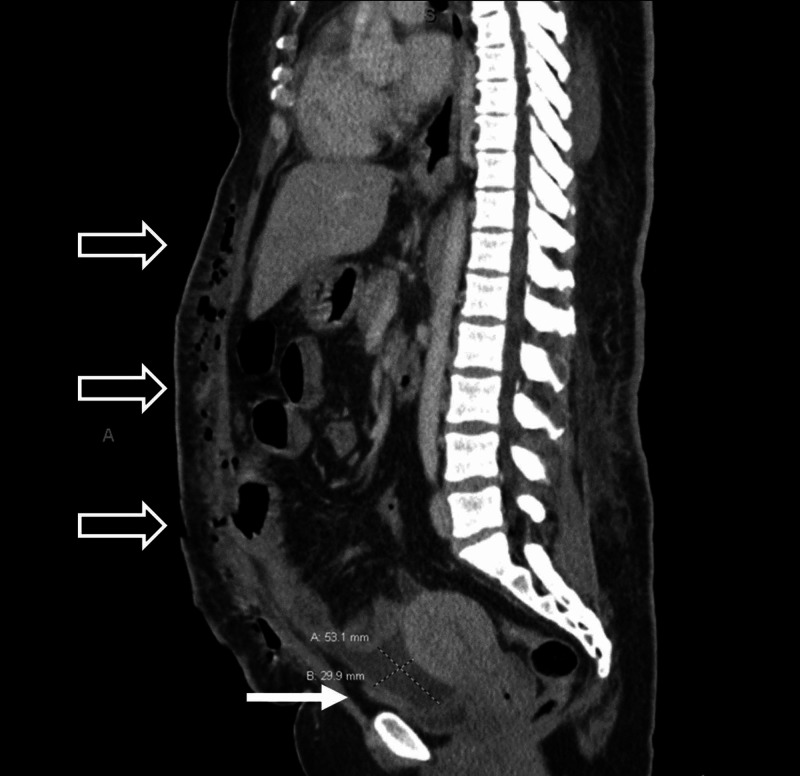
Sagittal CT image showing an intrapelvic fluid collection anterior to the uterus measuring approximately 5.3 x 3.0 x 6.9 cm (white arrow). Numerous foci of subcutaneous emphysema can also be seen (blank arrows).
